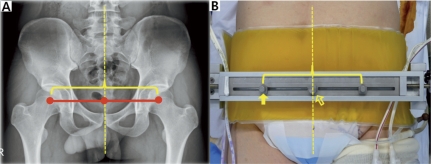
A. The distance between femoral head centers on both sides was measured on a radiograph of the pelvis. B. A customized metal ruler with two mobile pegs was developed to replicate the inter-femoral head distance. Dotted line: pelvic midline; empty arrow: center rod; solid arrow: mobile peg (landmark X).
